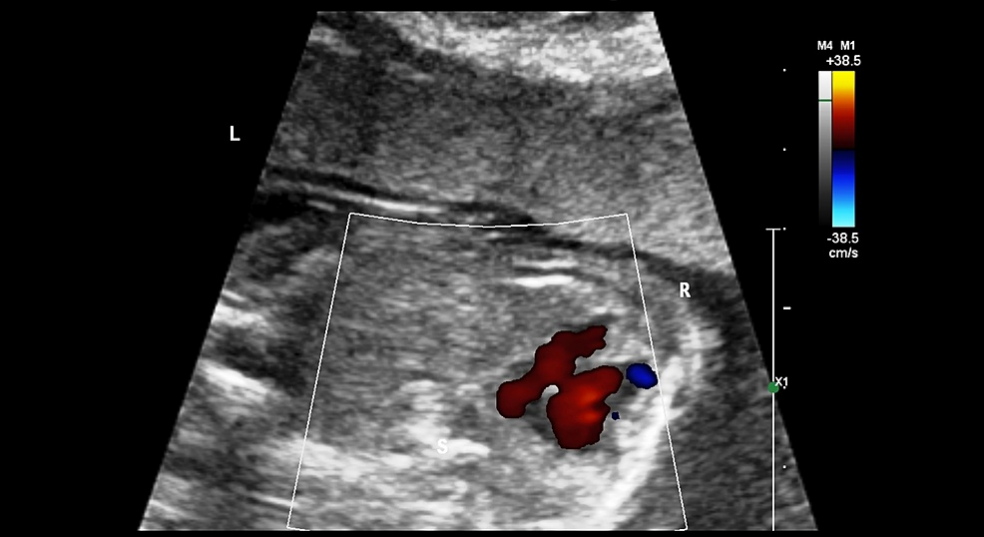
Color Doppler showing the right to left shunting of blood through the ventricular septal defect.
